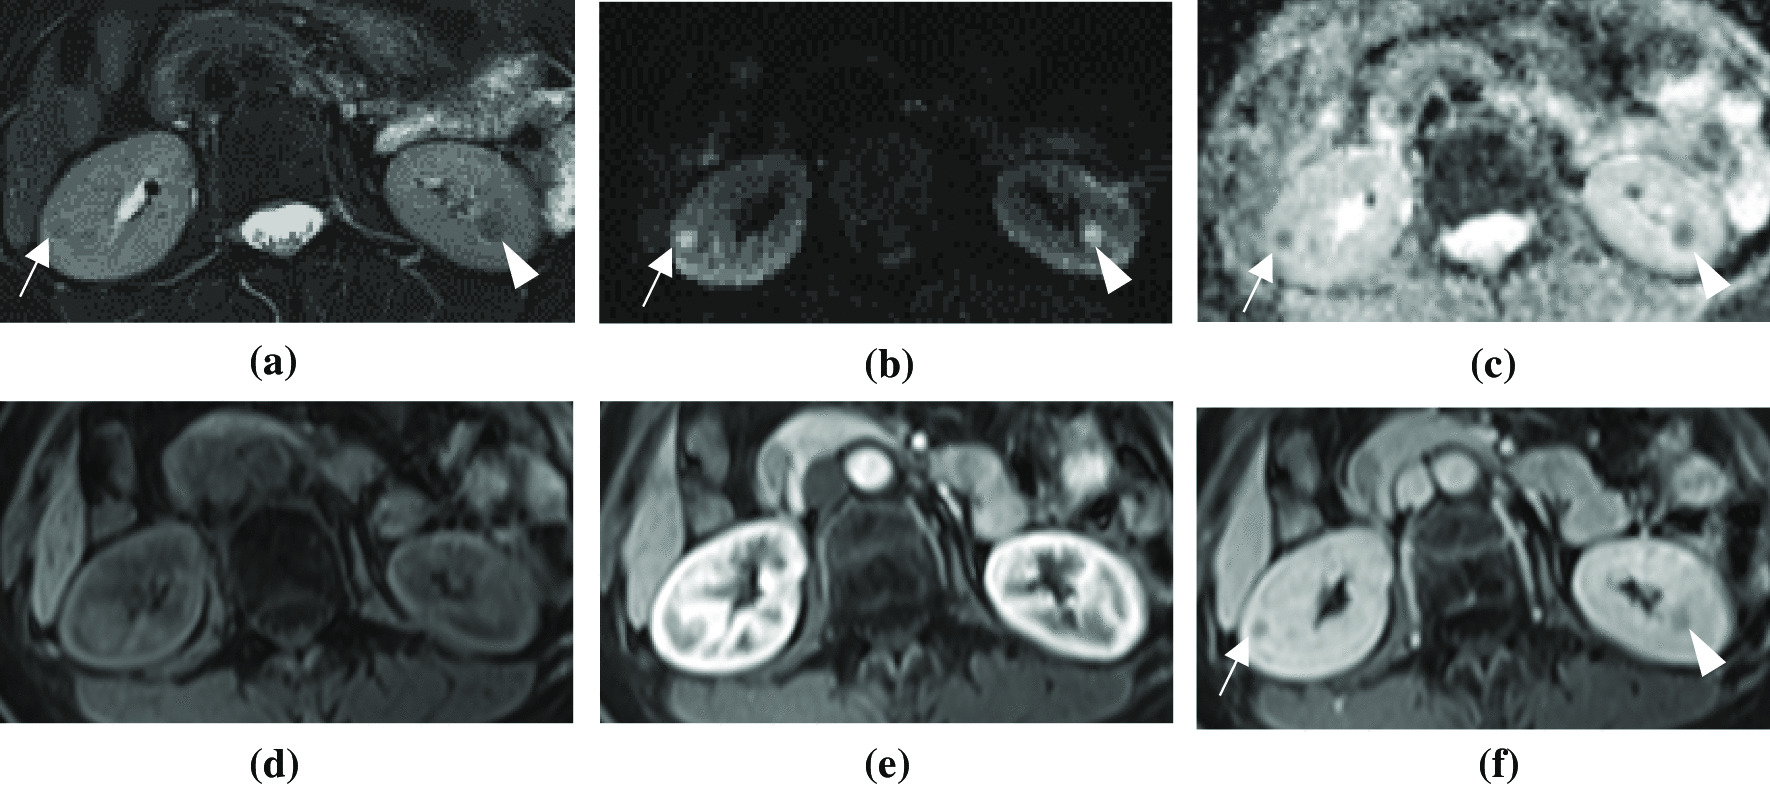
Abdominal MRI with contrast enhancement performed in January 2010. a On axial fat-saturated T2-weighted images, papillary adenomas present as well-defined hypointense nodules (arrow and arrowhead) in the bilateral renal parenchyma. b, c Papillary adenomas present high signal intensity (arrow and arrowhead) on axial diffusion-weighted (*b*-value: 800) images and low signal intensity (arrow and arrowhead) on the corresponding apparent diffusion coefficient maps, suggesting diffusion restriction. d, e, f On axial dynamic contrast-enhanced T1-weighted images with fat suppression, papillary adenomas present isointensity in the renal parenchyma on unenhanced images and relatively poor contrast enhancement in the arterial and venous phases (arrow and arrowhead)
